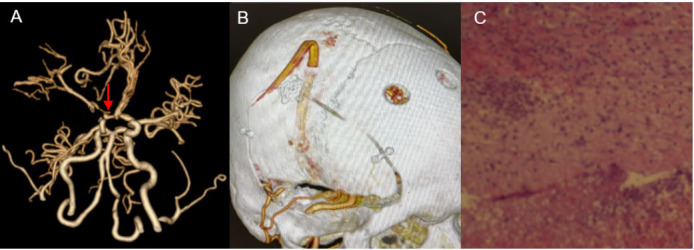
Postoperative imaging manifestations of our hospital. (A) CTA
